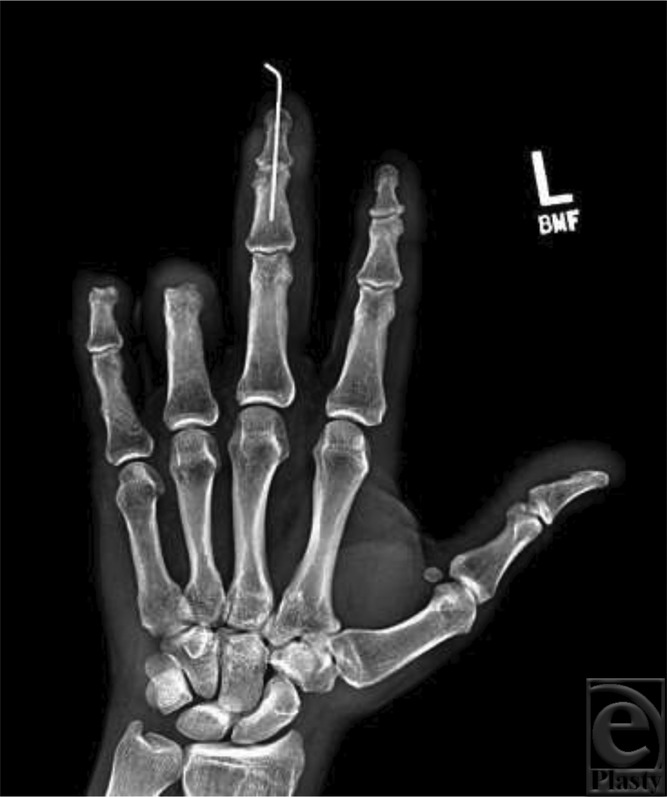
Postoperative radiograph demonstrating soft-tissue coverage of the amputation stumps of the small fingertip and the ring finger at the PIP joint. Also shown is skeletal fixation of the long finger DIP joint for treatment of the mallet deformity. PIP indicates proximal interphalangeal; DIP, distal interphalangeal.
